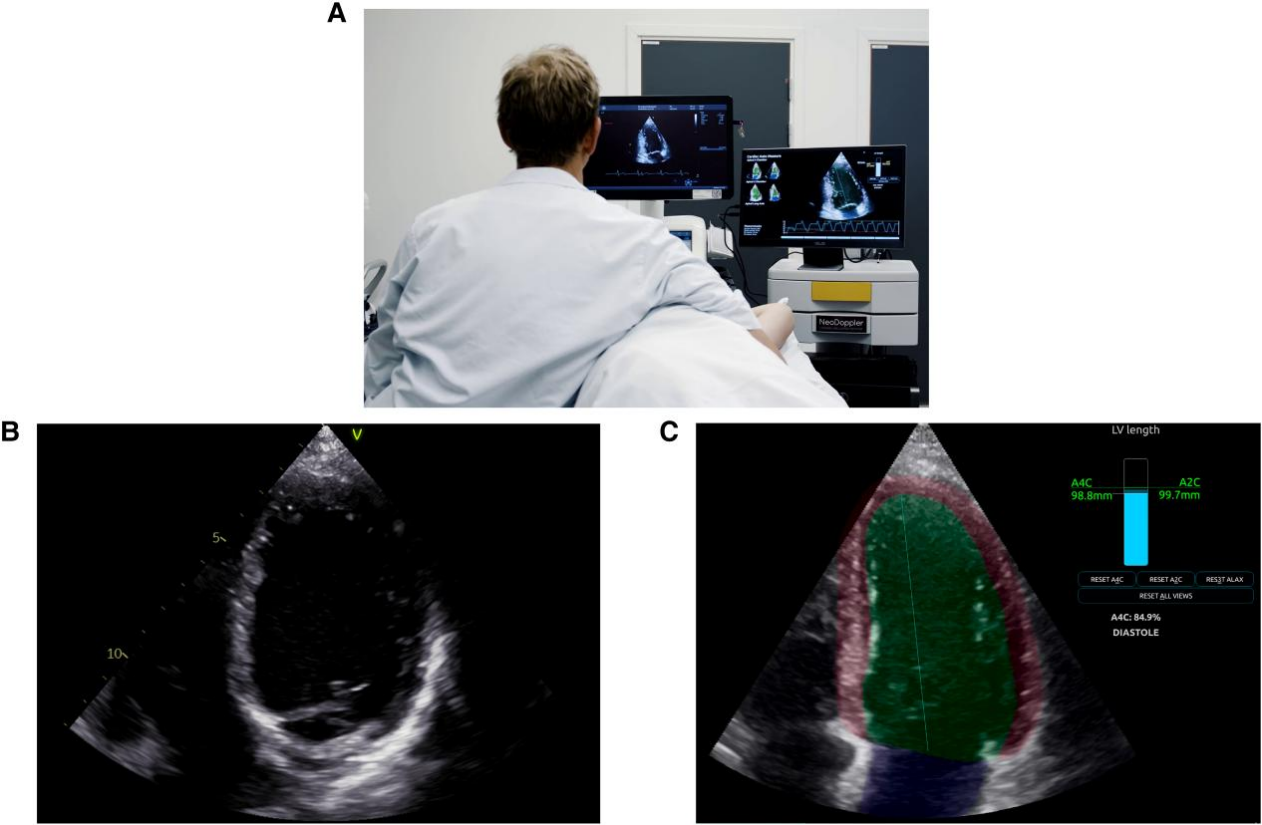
The real-time deep-learning feedback of view-specific left ventricular length and apical foreshortening. (*A*) The real-time DL-guiding tool (right screen) adjacent to the ultrasound machine (left screen). The tool is also visualized in Supplementary data online, *Video S1*. (*B* and *C*) Screenshots of the DL real-time foreshortening detection from four-chamber (A4C) recordings of the same patient. (*B*) Foreshortened A4C. (*C*) A4C recording using the DL-guiding tool without foreshortening (no significant difference between the A4C LV length and corresponding A2C LV length in the LV length indicator to the right in the image).
